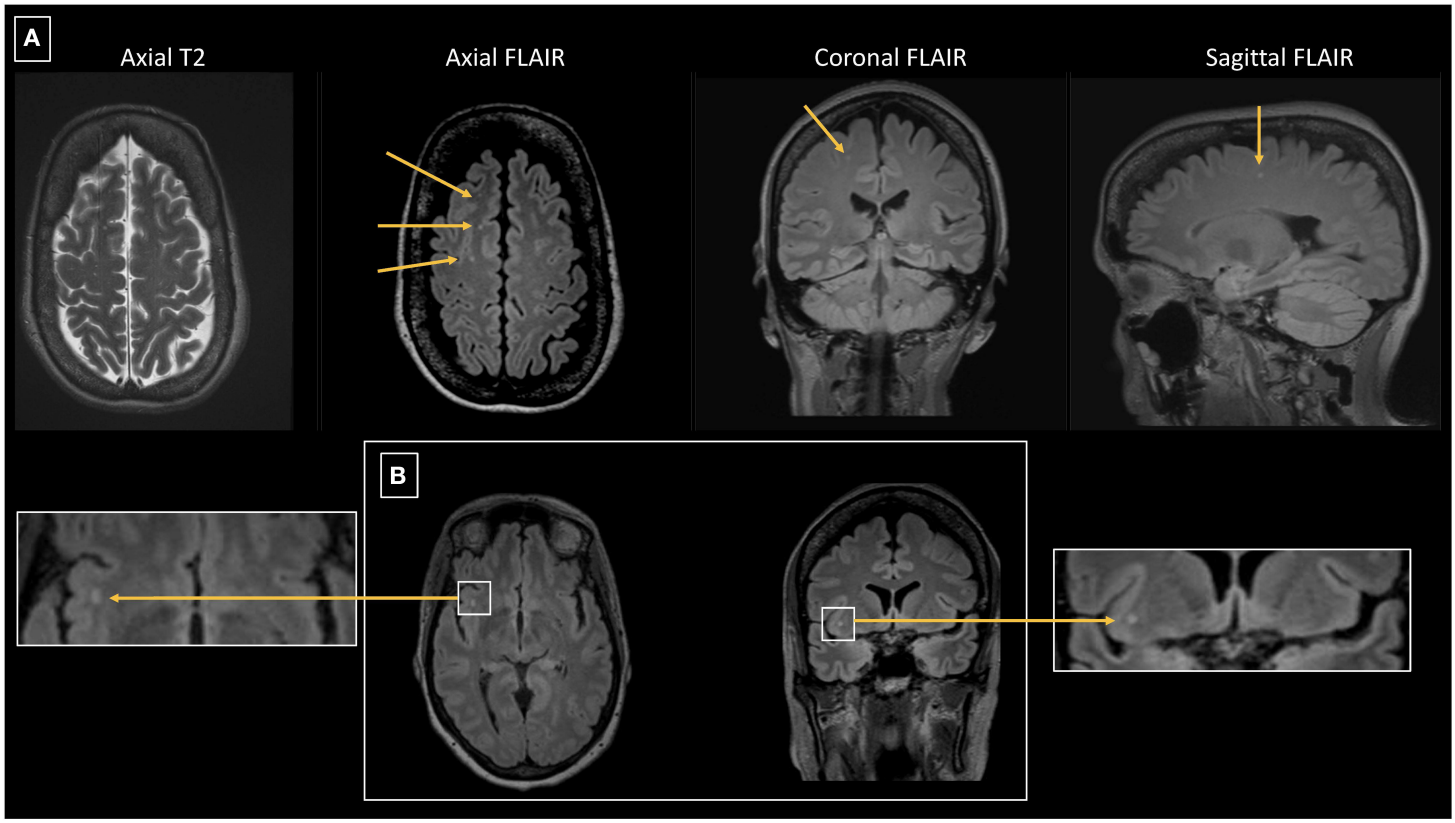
(A) 64 year-old female with episodic migraine with aura who averaged 5 migraine attacks per month. No history of vascular risk factors. Axial FLAIR images show 3 of 9 right frontal white matter lesions all of which are small and punctate in contour. Lesions are less conspicuous on axial T2 images than seen on FLAIR images. One of the lesions is depicted on coronal and sagittal FLAIR images. (B) Axial and coronal FLAIR images in the same patient show a punctate lesion in the right insular white matter.
